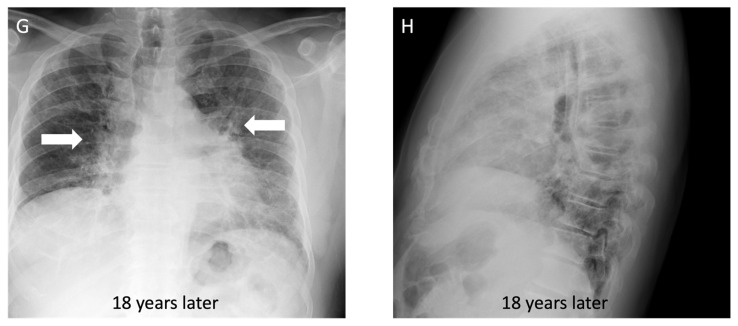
A 43-year-old non-smoking male with 15-year history of tungsten carbide exposure as a metal grinding tool maker, diagnosed with hard metal lung disease, which was confirmed with surgical lung biopsy. (A,B) Axial computed tomography images demonstrate patchy ground glass opacities (arrows) and small centrilobular nodules (white circle) with minimal architectural distortion and traction bronchiectasis; (C,D) computed tomography images 18 years later demonstrate long-term evolution of fibrosis, with development of traction bronchiectasis (white circle) and mild honeycombing (black arrows) on the background of ground glass opacity and coarse reticulation; (E) posteroanterior view and (F) lateral view chest radiographs show a perihilar and lower lung zone predominant reticular abnormality (black arrows); (G) posteroanterior view and (H) lateral view chest radiographs 18 years later demonstrate a reduction in lung volumes, and the prior interstitial opacities appear coarser and more prominent, particularly in the perihilar lung (white arrows).
